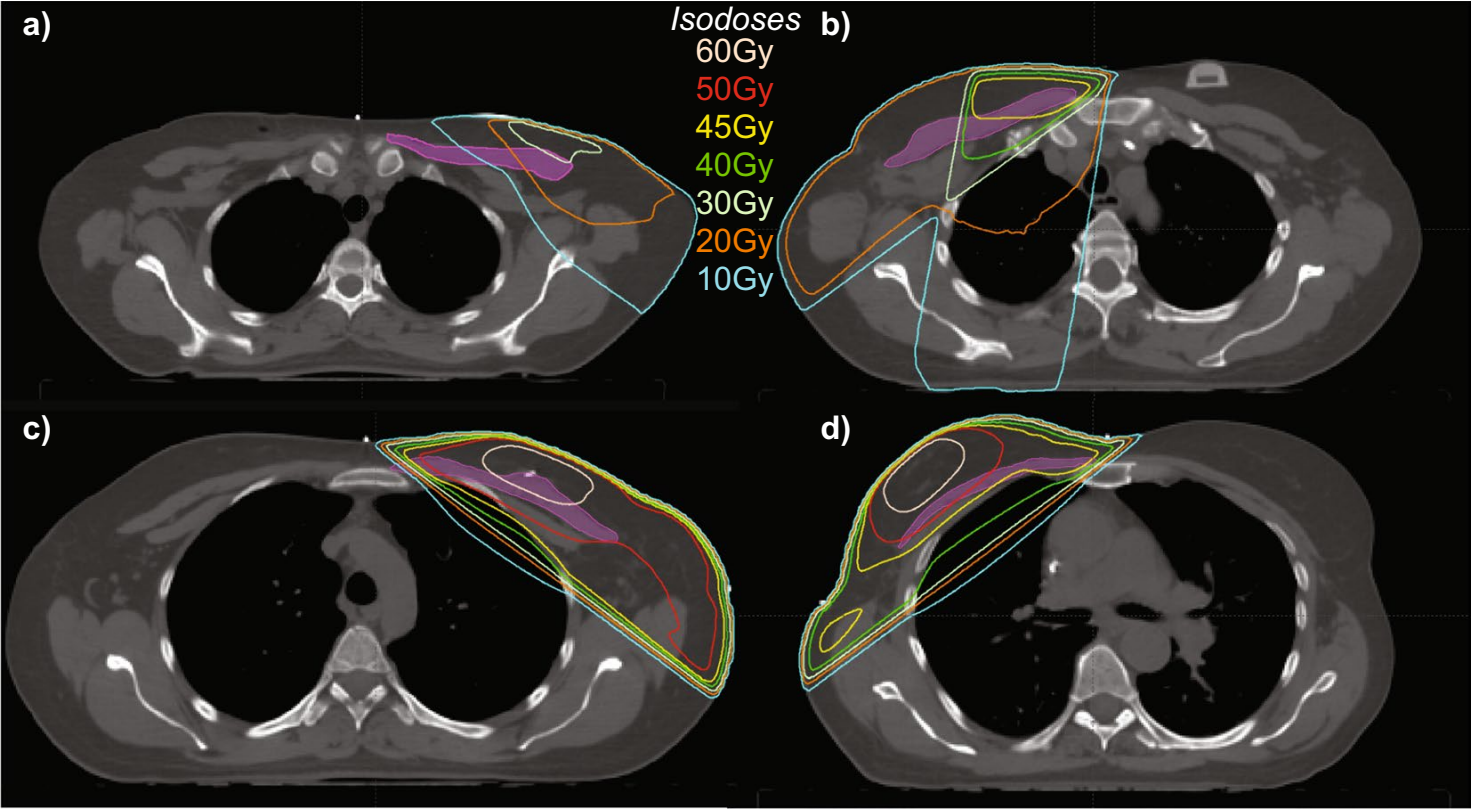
The radiation treatment plan for two representative patients are shown as isodose lines superimposed on axial computed tomography slices acquired at the T3 (a,b) and T6 (c,d) vertebrae. The treatment plans highlight differences in the amount of radiation delivered to the pectoralis major muscle (pink shaded region) differ between a breast cancer patient undergoing RT with 2 fields (A,C) and a patient undergoing RT with 3 fields (b,d).
